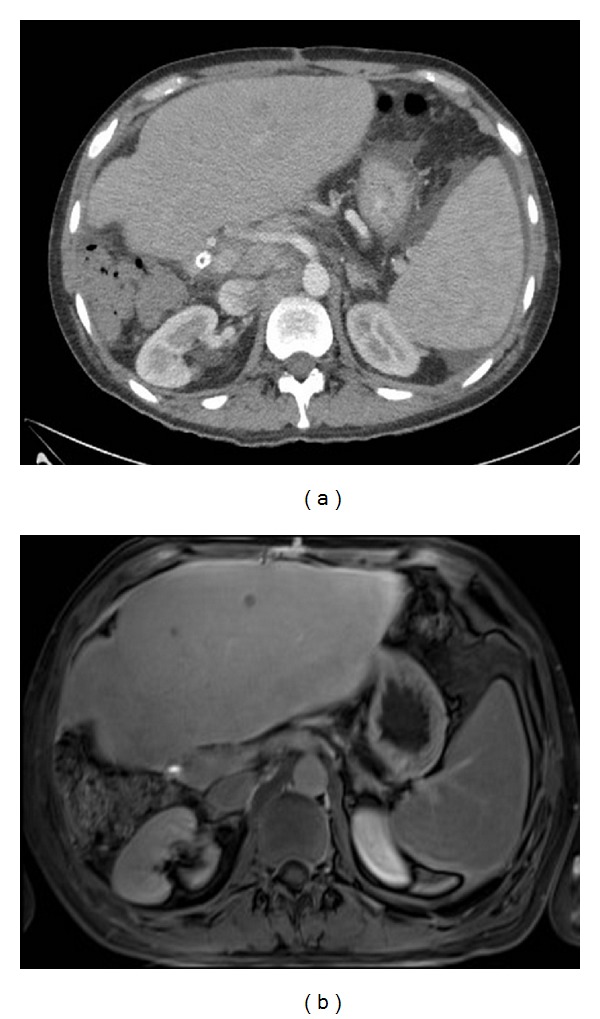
Contrast CT compared with Gd-EOB-DTPA-enhanced MRI. 65 y. male after right hepatic lobe resection. Routine follow-up CT scan (a) shows subtle low attenuation lesions in the left hepatic lobe, clearly seen on MRI with Eovist (b).
